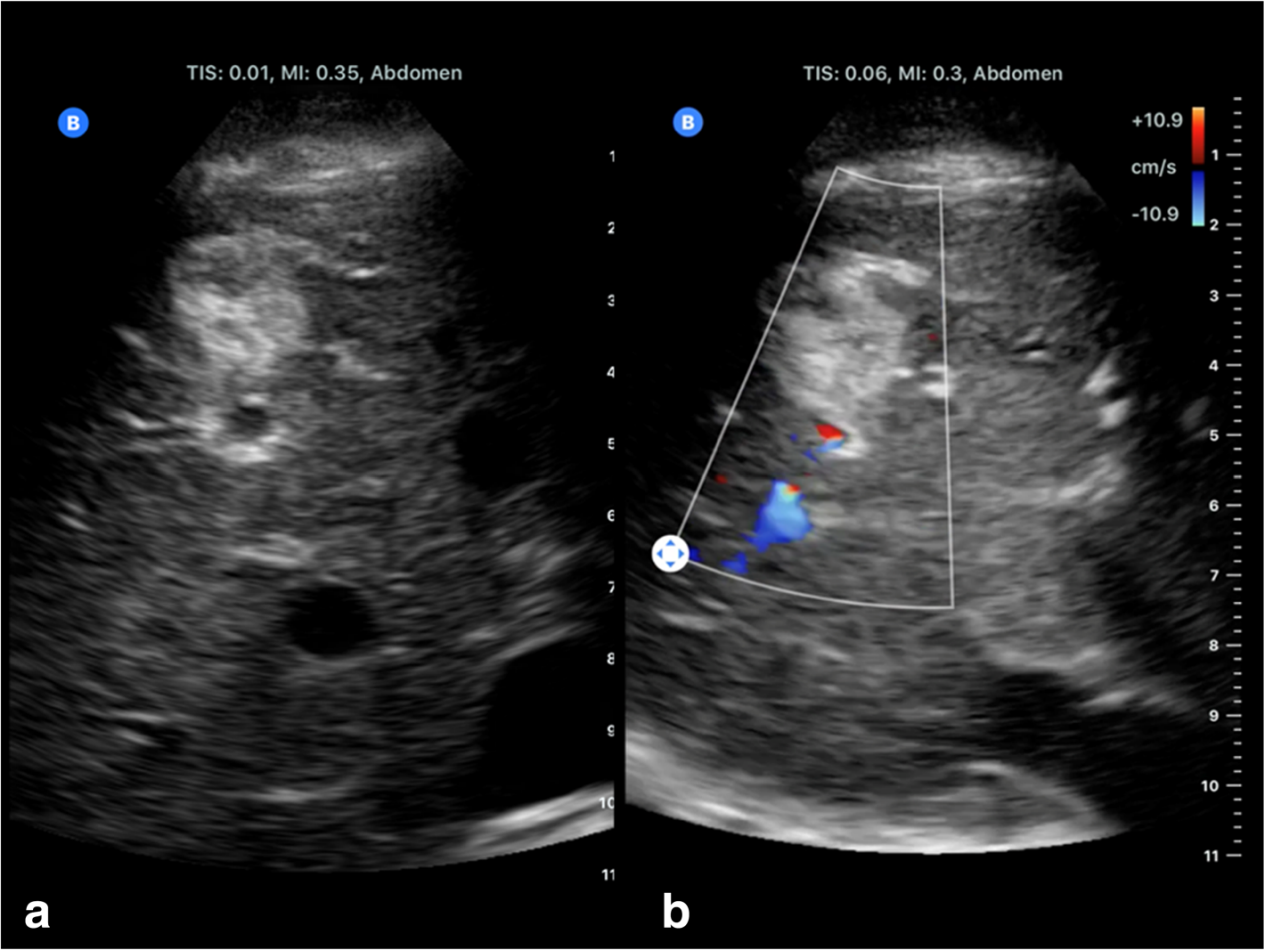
Butterfly’s iQ emulate curved transducers: B-scan ultrasound (a) and colour Doppler (b) of a liver haemangioma
